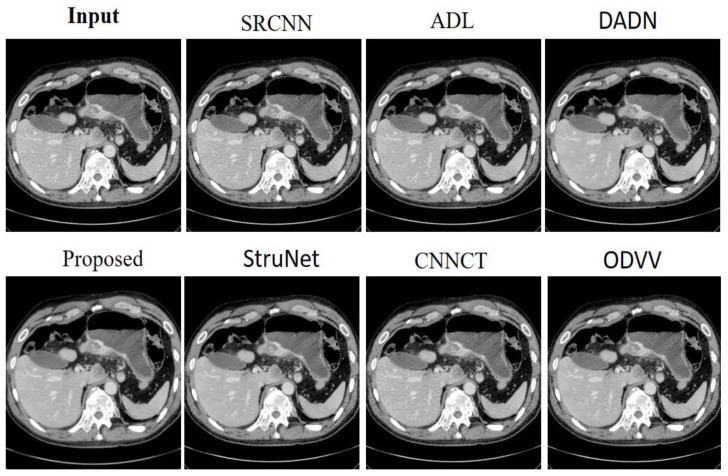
Outcomes derived from assessing noise-reduction algorithms on the Abdomen CT dataset.
